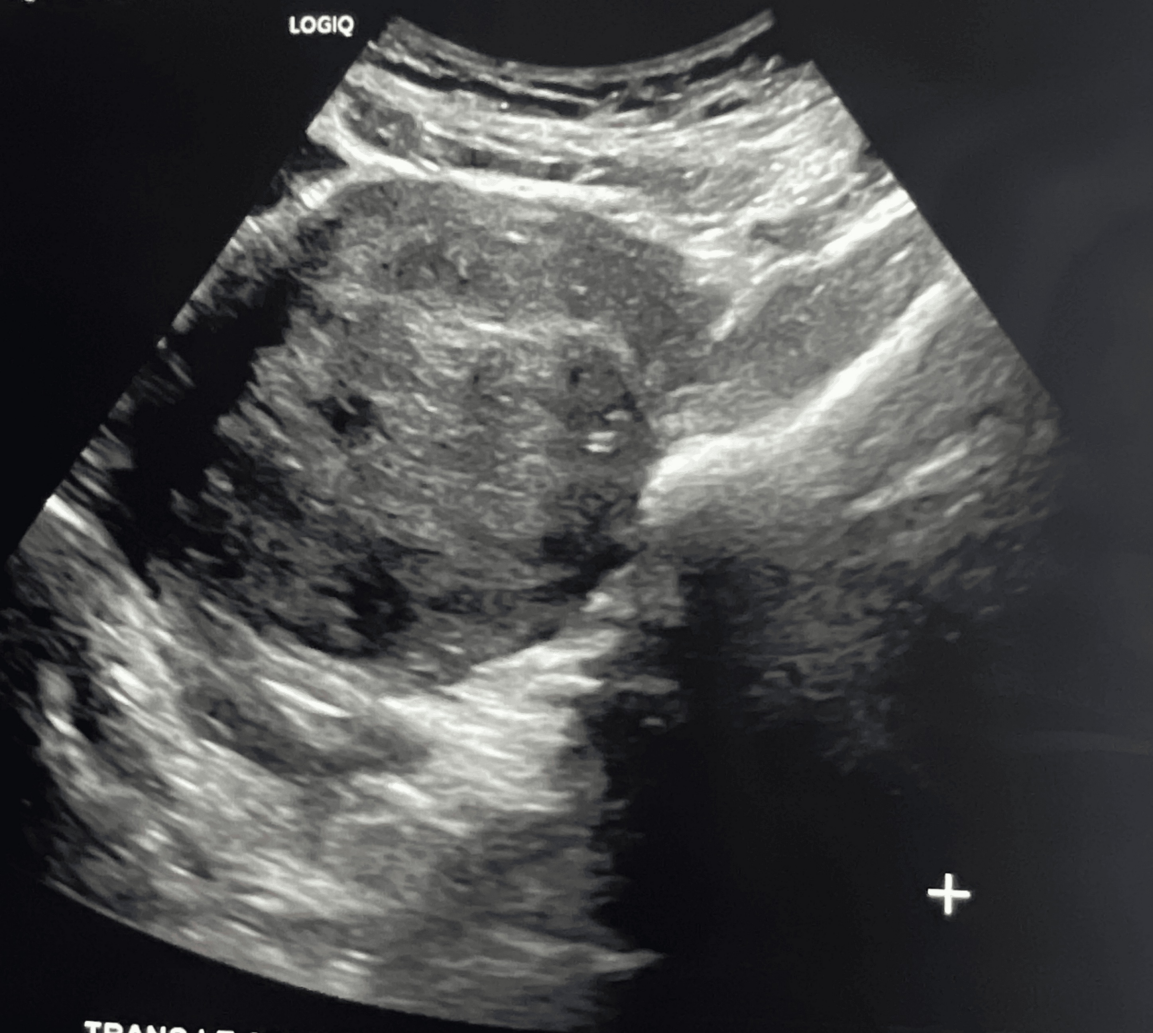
Ultrasound showing fumarate hydratase (FH)-deficient leiomyoma in the lower uterine section (paracervical)
